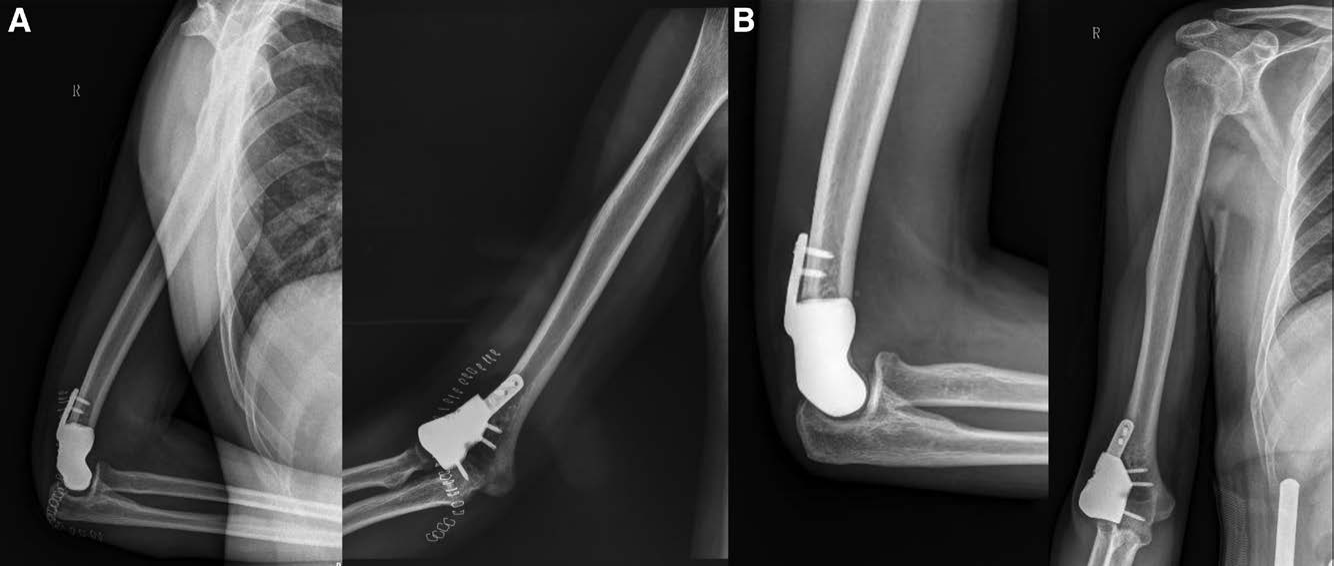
X-rays results of follow-up after operation. (A) Anteroposterior and lateral X-rays one week after surgery, (B) anteroposterior and lateral X-rays six months after surgery.
